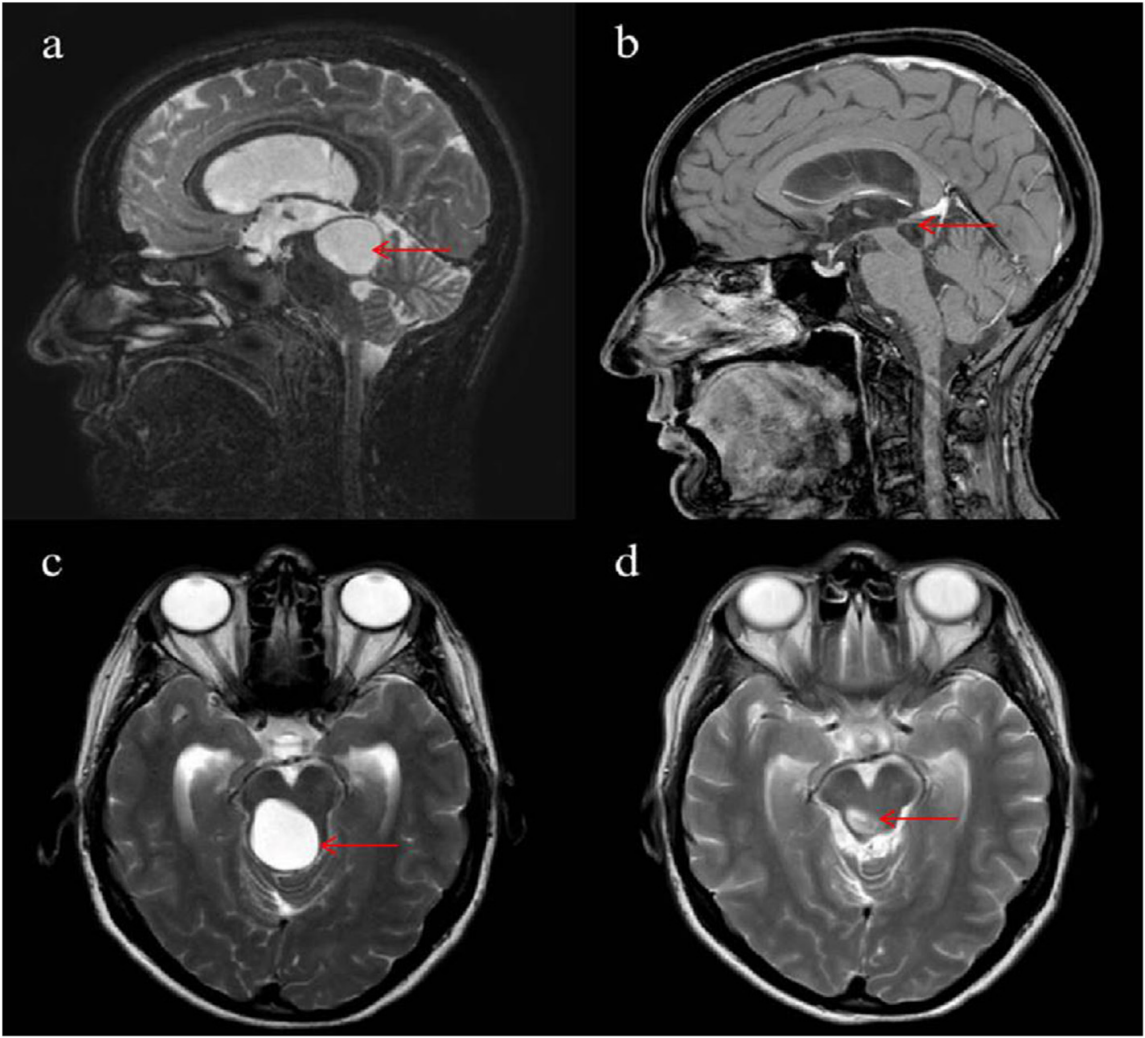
Brain MRI of case 1. Preoperative sagittal (a) and axial (c) T2-weighted MRI. Postoperative sagittal T1-weighted (b) and axial T2-weighted (d) MRI.
